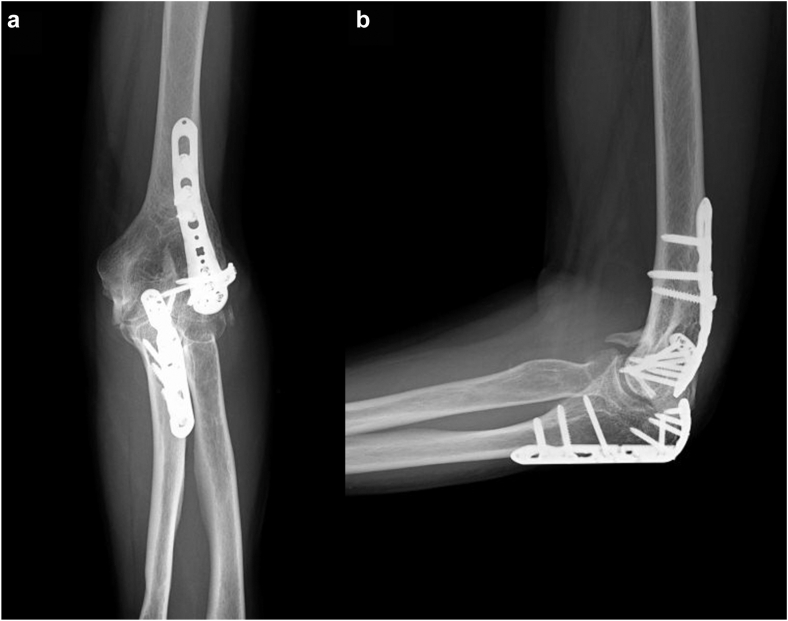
Plain radiographs before resection of heterotopic ossification. (a) Anteroposterior radiograph, (b) lateral radiograph.
